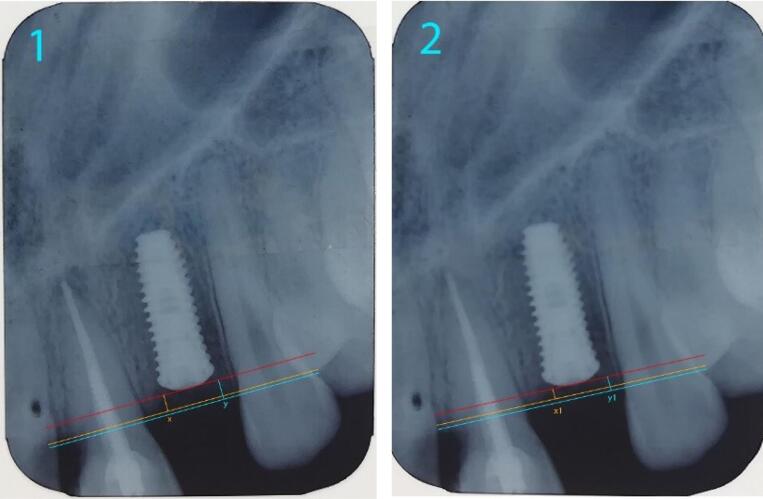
The amount of bone resorption was calculated by two experienced oral and maxillofacial radiologists (not the operator) blinded to the technique, using the software to identify the smallest pixel identifiable from the bone, according to the patient’s radiographs. In case of disagreement, the examination of a third examiner was recorded as the treatment outcome.
